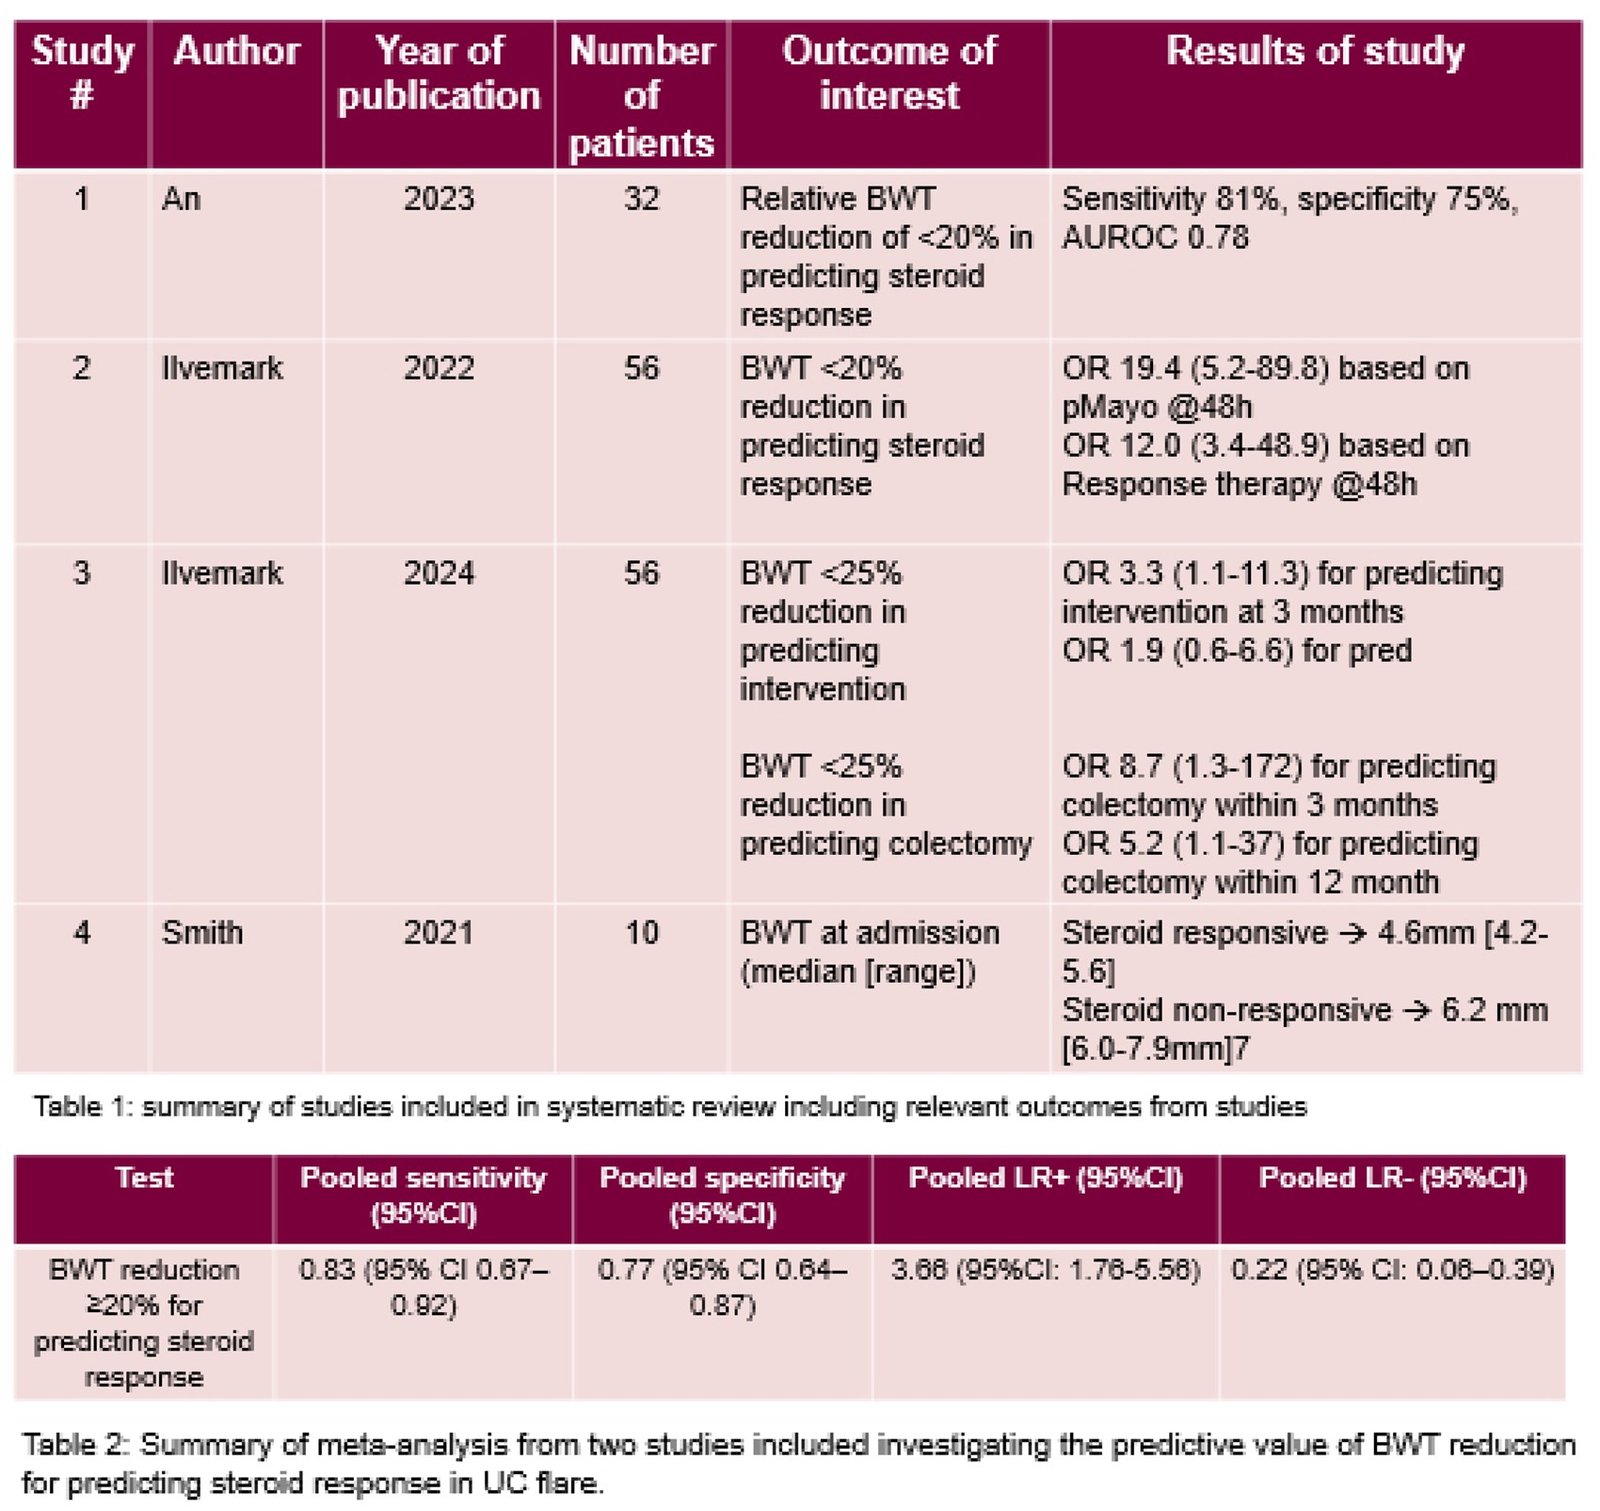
# Poster Session II – Poster of Distinction II - A209 PREDICTIVE VALUE OF INTESTINAL ULTRASOUND FOR TREATMENT RESPONSE AND LONG-TERM OUTCOMES IN SEVERE ULCERATIVE COLITIS: A SYSTEMATIC REVIEW
